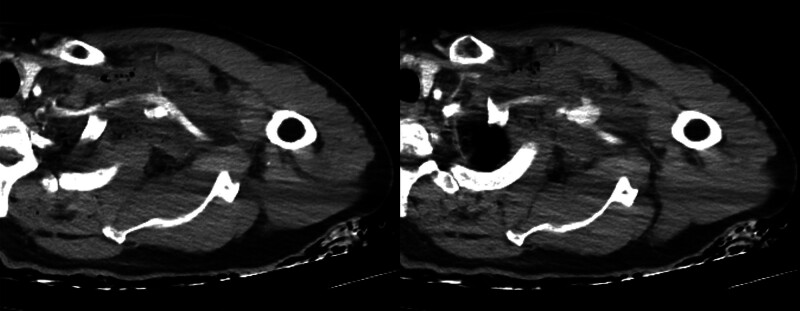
CT angiography showing extravasation of the left subclavian artery. CT = computed tomography.
